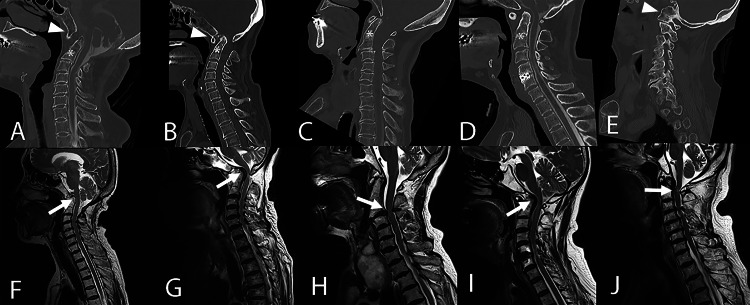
Preoperative CT or CT myelography (upper row) and magnetic resonance imaging (lower row) scans. (A, F) Case 1, (B, G) Case 2, (C, H) Case 3, (D, I) Case 4, and (E, J) Case 5. White arrow heads: C1 assimilation. Asterisks: C2-C3 vertebral fusion.  White arrows: spinal cord compression at adjacent mobile segments. CT: computed tomography.
